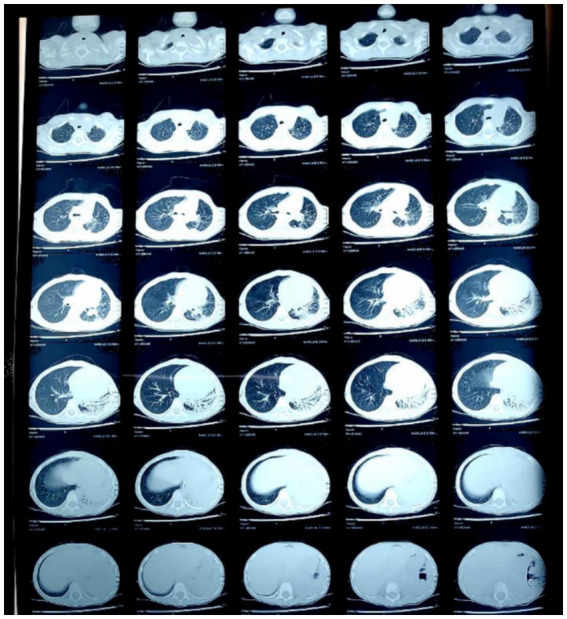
Post expulsion high resolution computed tomography showing little or no signs of consolidation.
